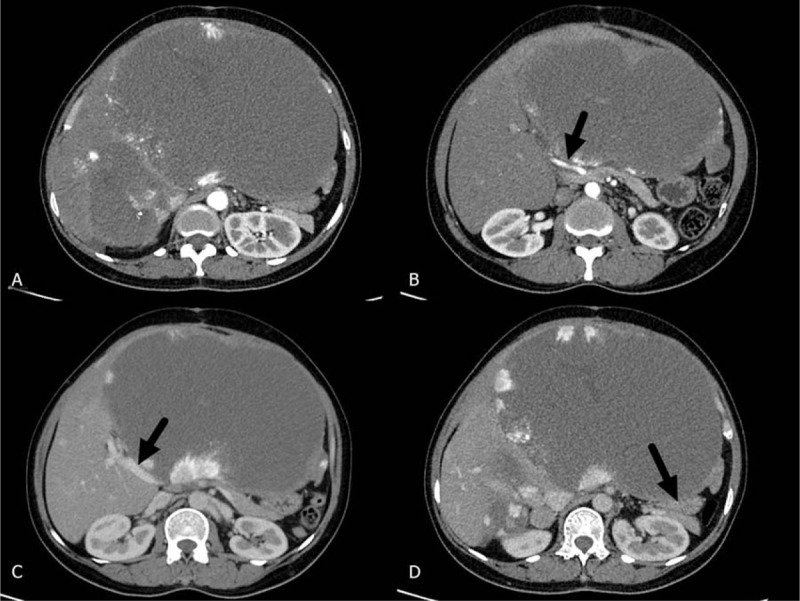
CT images of a patient with Kasabach-Merritt syndrome associated with giant liver hemangioma. (A) Multiple liver hemangiomas, with the larger one in the left liver; (B) compression of the hepatic artery (black arrow); (C) compression of the portal vein (black arrow); (D) the gastric outlet is obviously compressed (black arrow).
